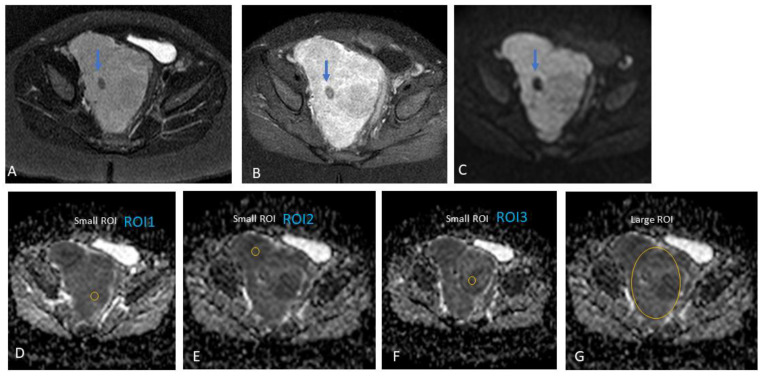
Presacral neuroblastoma in a one-year-old boy. Heterogeneous tumour, predominantly solid. Note should be made of the small focal area of low signal intensity on T2 (A), post-contrast T1 (B), and diffusion-weighted (DW) trace (C) MR images (arrows), which could represent a focal area of calcification or fibrosis. This focal area was avoided during the placement of small regions-of-interest (ROIs) within the tumour during data analysis. (A) Axial T2, (B) axial post-gadolinium T1, and (C) axial DWI (b-value: 600 mm^2^/s). (D–F) Three small ROIs on the ADC map with an area of 17.6 mm^2^ and an average value of 0.78 × 10^−3^ mm^2^/s, and (G) one large ROI with an average area of 1280.2 mm^2^ and an average value of 0.93 × 10^−3^ mm^2^/s.
